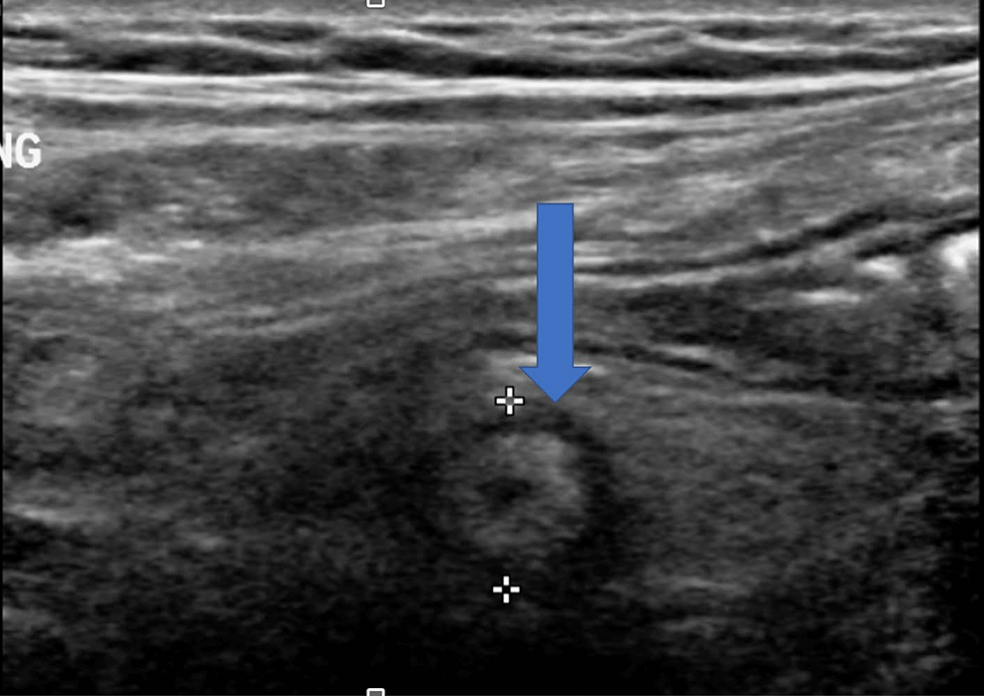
Transverse image of the right inguinal area ultrasound done on June 22nd.
